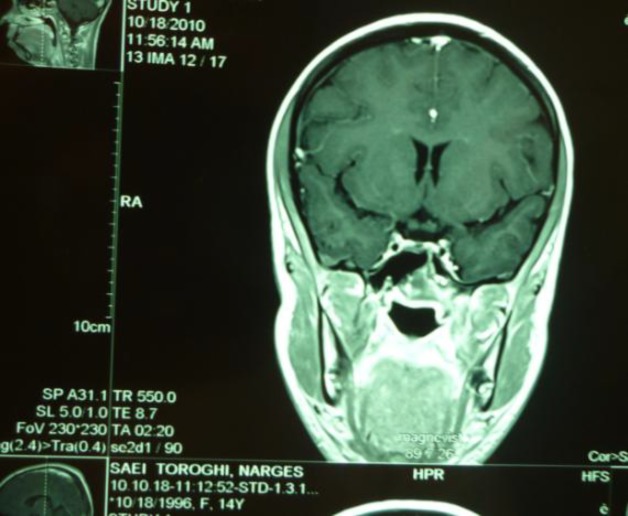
T1 MRI Showing significant resolution of the tumor after radiotherapy.There is only small soft tissue mass in the nasopharynx area, without parapharyngeal involvement
